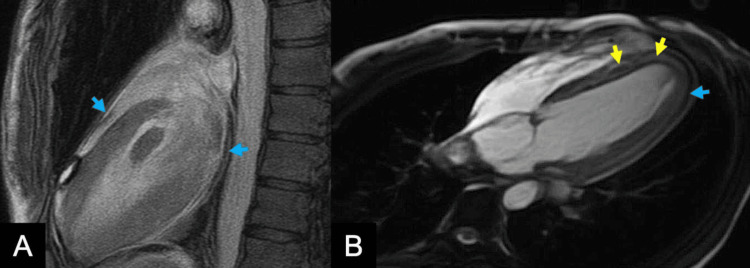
CMR with sagittal view (A) and transverse view (B) with LGE showed multifocal small areas of patchy subendocardial and mesocardial enhancement, involving basal, mid segments, and distal segments (yellow arrows). The pericardium is thickened, edematous, and enhancing with minimal pericardial effusion noted (blue arrows). CMR: Cardiac magnetic resonance imaging; LGE: Late gadolinium enhancement
